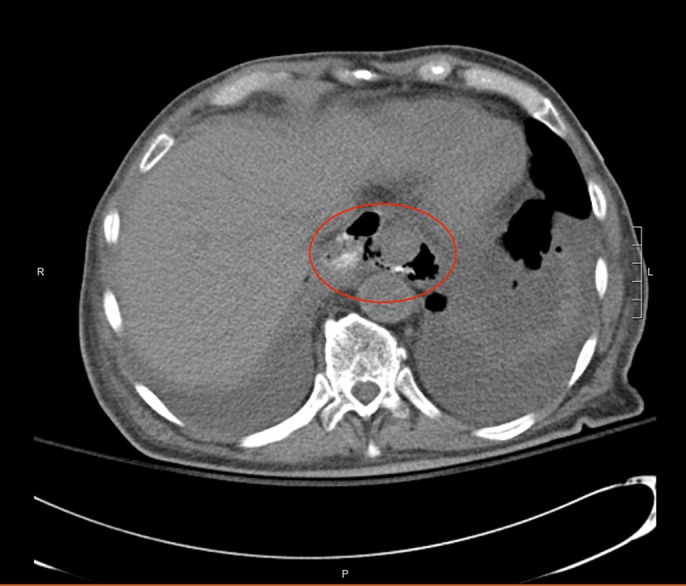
Chest computed tomography with oral and without intravenous contrast on admission. The circled area designates foci of extraluminal air adjacent to the distal esophagus tracking to the pleura or medial left lower lobe.
